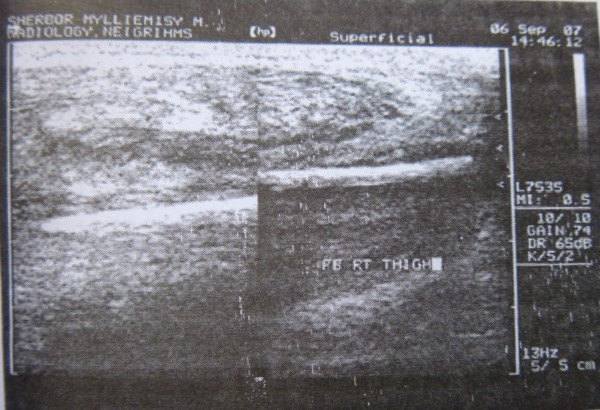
Ultrasonographic picture clearly showing the foreign body.
